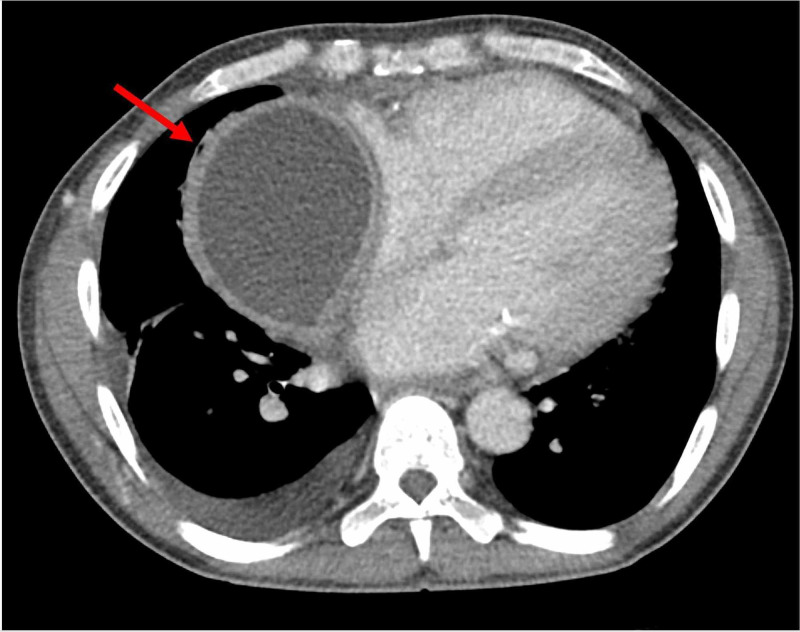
Pericardial Abscess: Axial CT Chest With IV Contrast.
